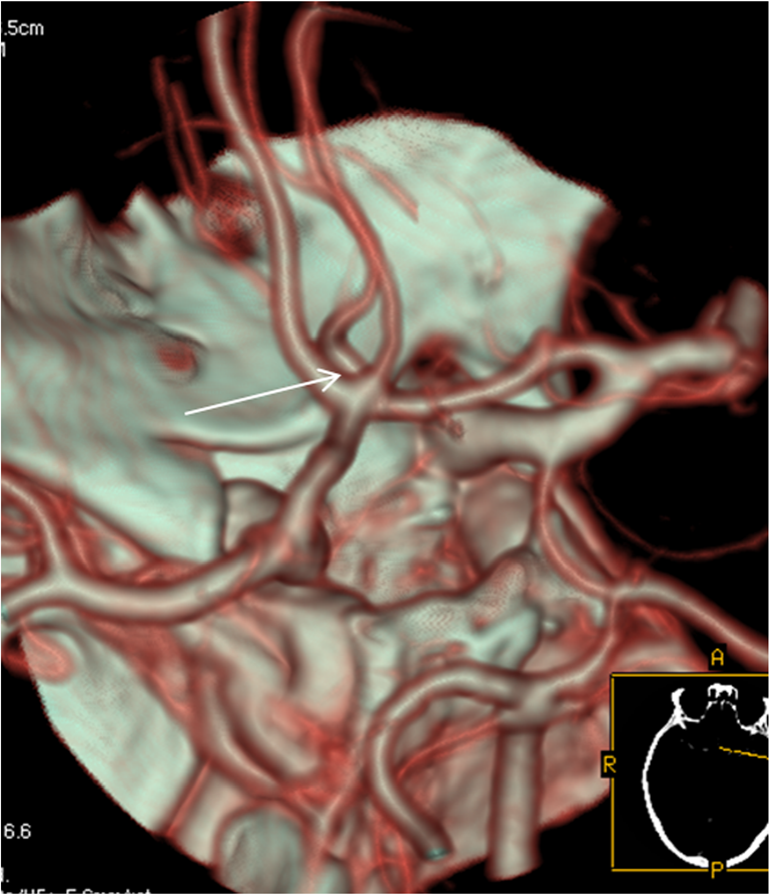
CTA (VRT 3D reconstructions) reveals the presence of a triplicated ACA (*white arrow*)
